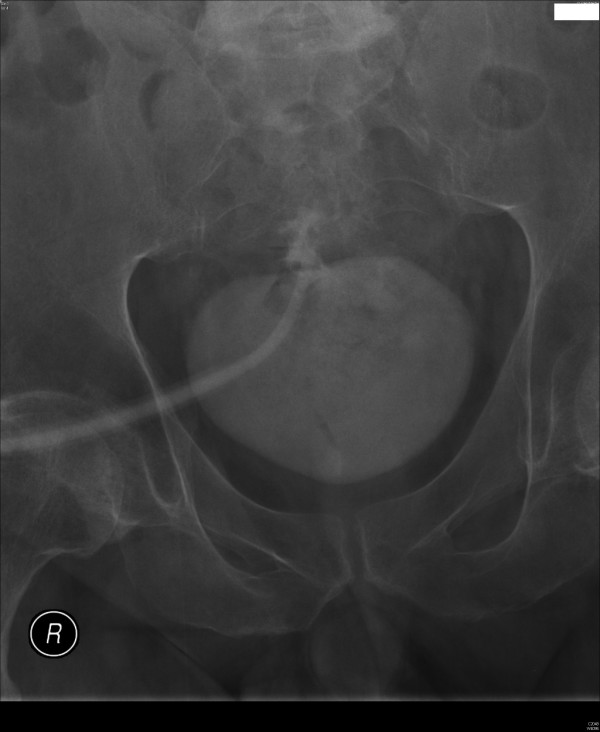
Another 20 ml of Optiray 300 was injected and X-ray was taken. This X-ray clearly demonstrated localised extravasation of contrast on the superior aspect of urinary bladder around the tip of Foley catheter.
